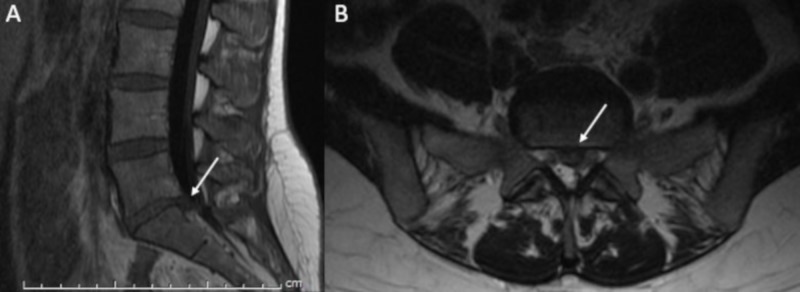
Case 1 diagnostic images. (A) Sagittal T1 FLAIR and (B) axial T2 weighted images of the lumbar spine demonstrate a left paracentral disk herniation with extruded disk compressing the left S1 nerve root. Arrows highlight areas of interest.
